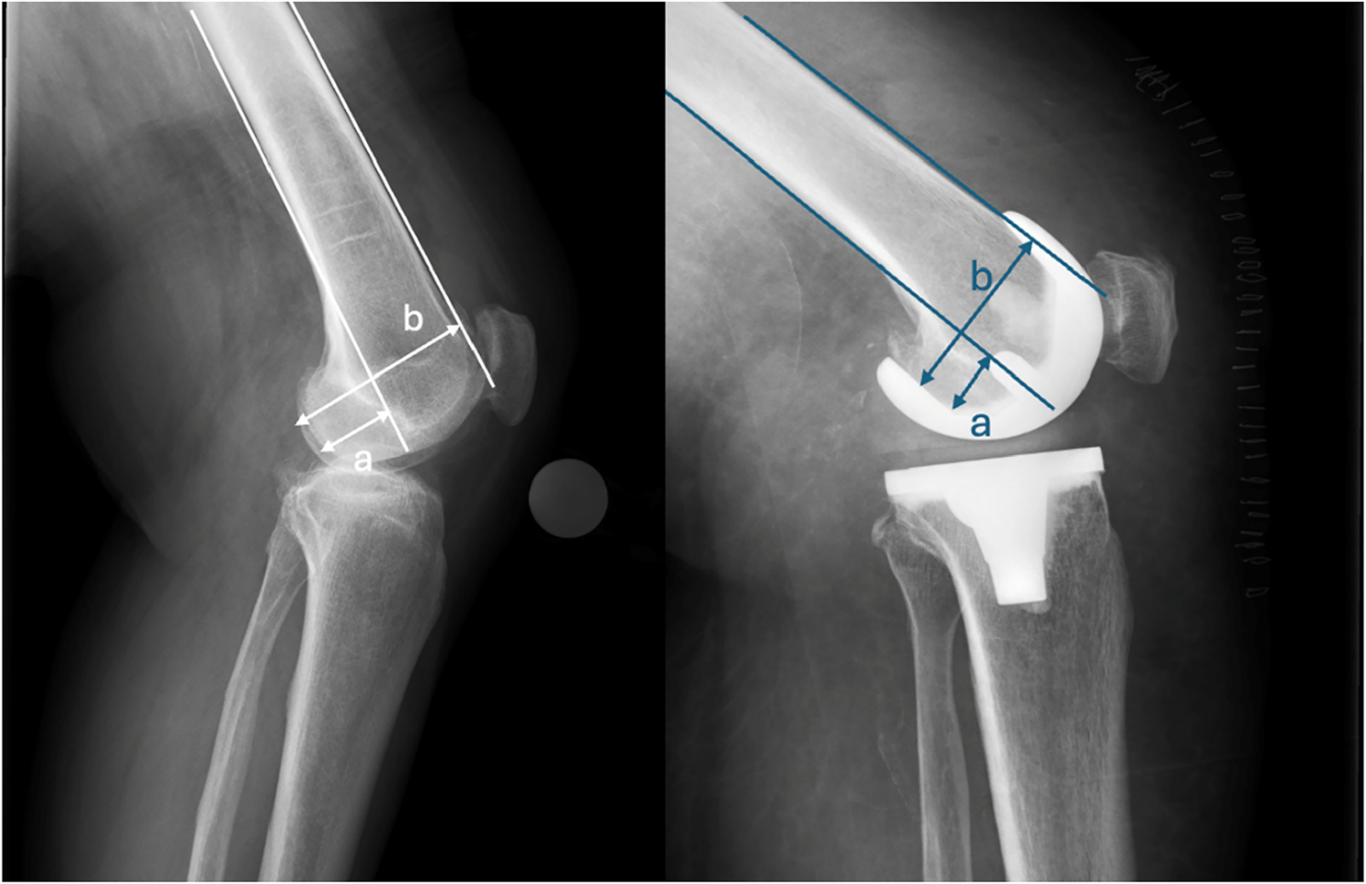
Measurement of posterior condylar offset (PCO) and posterior condylar offset ratio (PCOR) on true lateral radiographs. *a* = distance (mm) from a line tangent to the posterior femoral diaphyseal cortex to the posterior condylar margin; *b* = anteroposterior diameter (mm) of the distal femur at the same level; PCOR = *a*/*b*.
